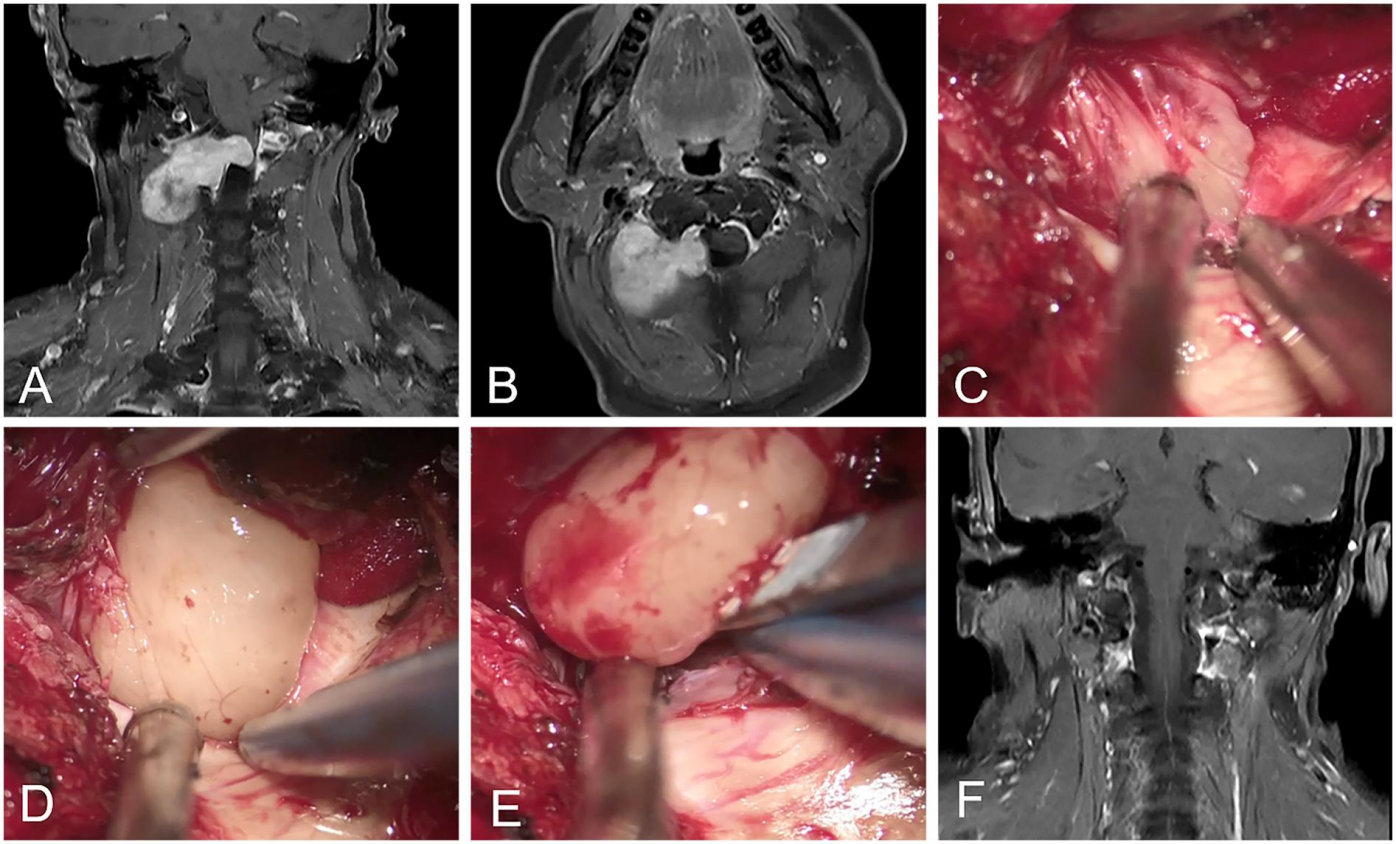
Representation of C1–C2 schwannomas. (A,B) The preprocedural MRI scan revealed the dumbbell-shaped schwannomas located in the right cervical spine at the C1–C2 intervertebral foramen. (C,D) The tumor was gently retracted laterally, and its interface with the dura mater was meticulously dissected. (E) The intradural tumor was successfully exteriorized via the trans-epidural approach. (F) Postprocedural MRI revealed no tumor residual.
